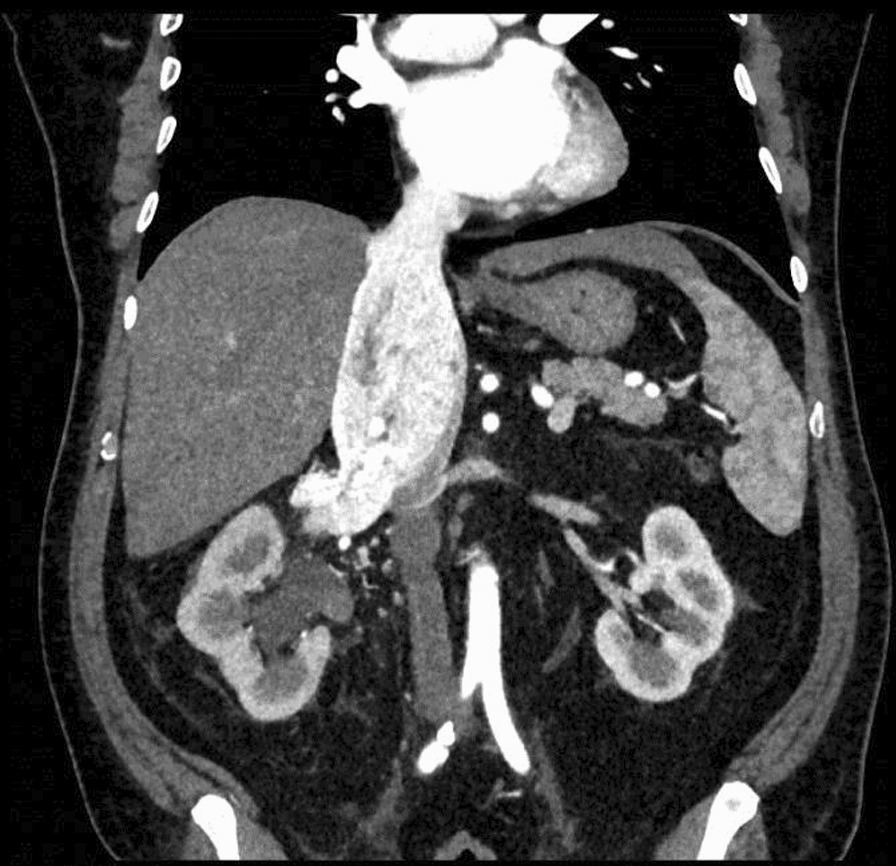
Preoperative computed tomography scan showing tumor mass and thrombus extension along inferior vena cava (coronal plane)
